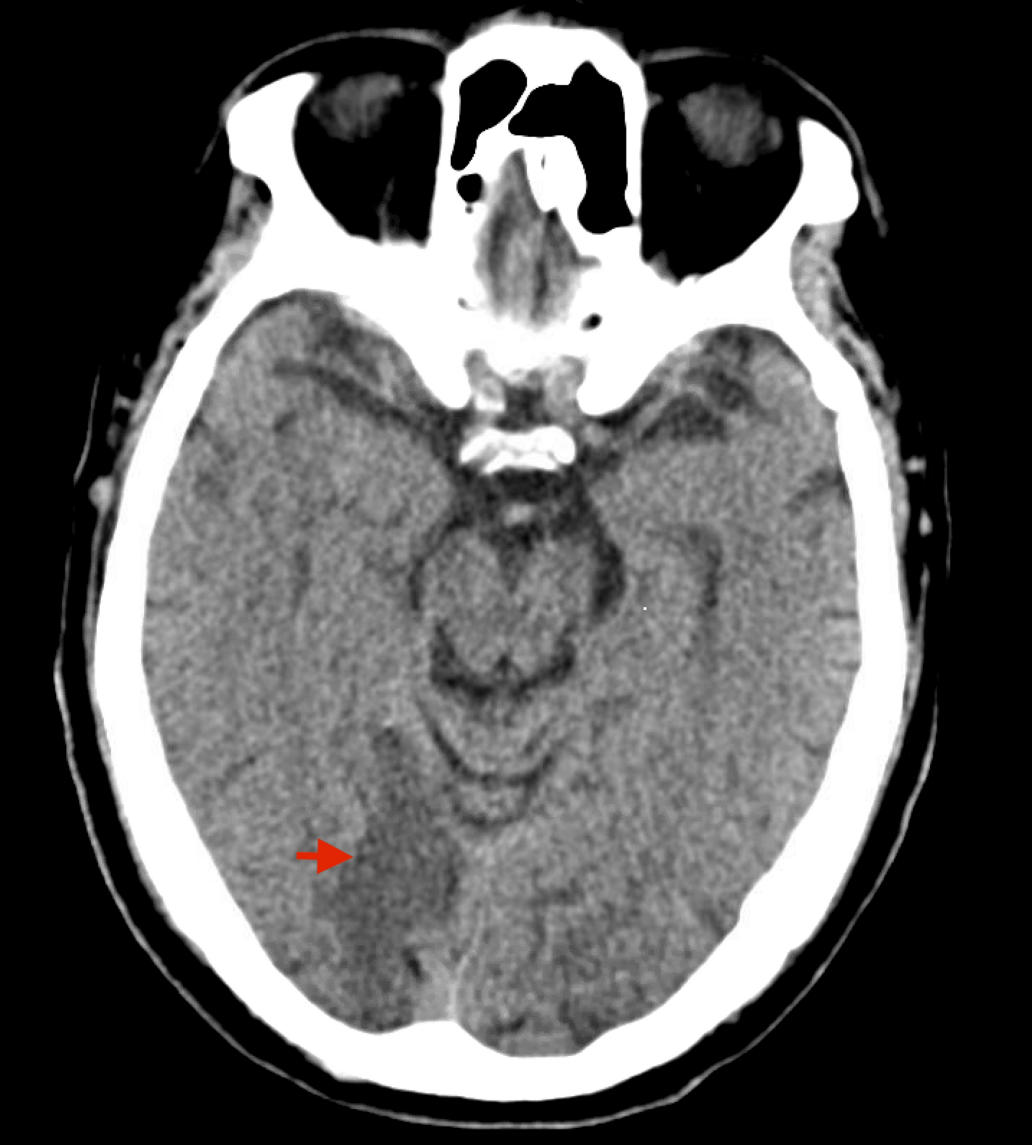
Head CT imaging Axial view of the CT scan of the head showing an area of hypo density in the right occipital lobe (red arrow) with loss of differentiation of gray and white matter consistent with the diagnosis of an acute stroke.
